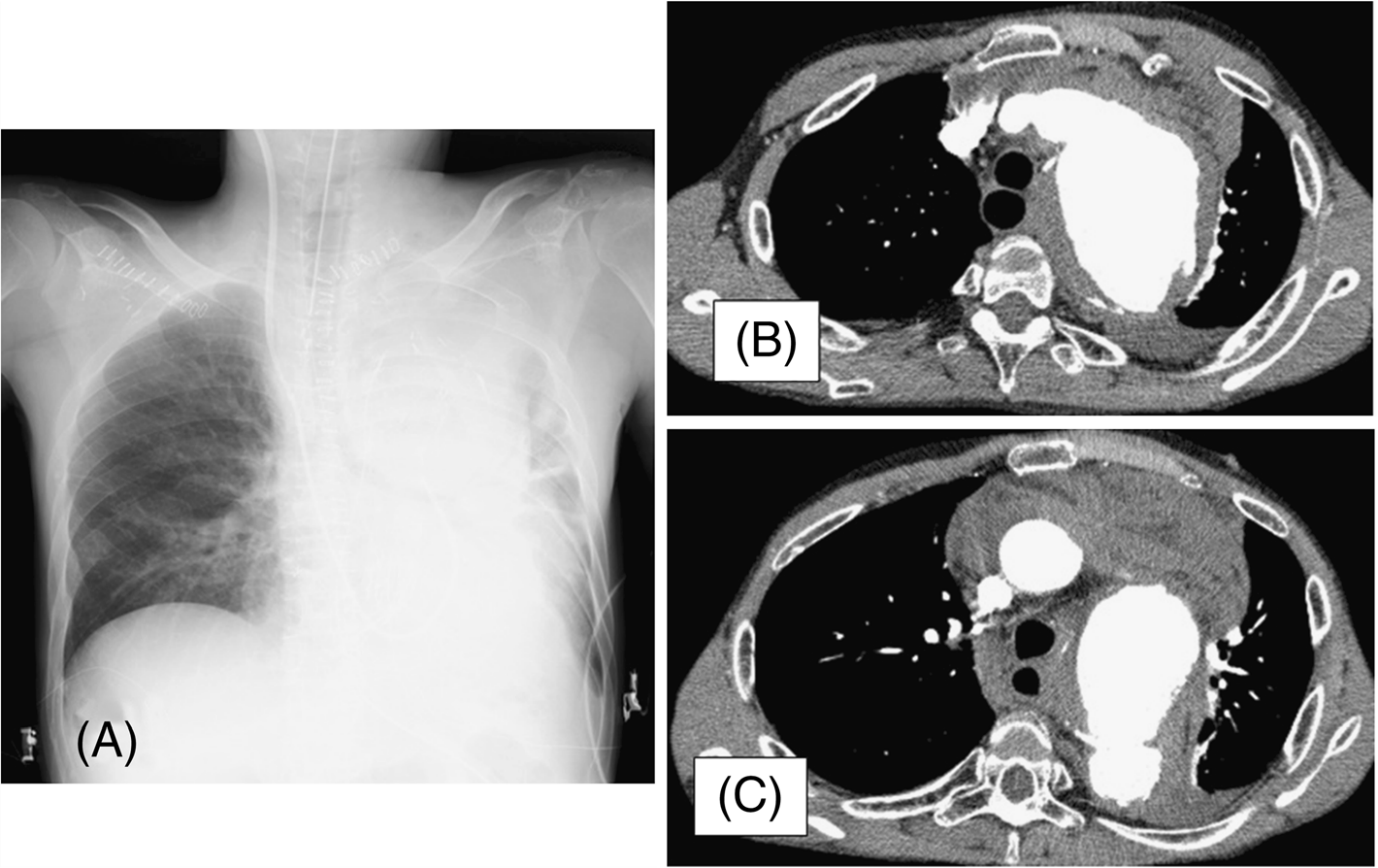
Chest radiograph and computed tomography scan of patient with GPA. (A) Chest radiograph of a patient with a very large aneurysm of the aortic arch. Evident are marked widening of the mediastinum and aortic contour. (B) and (C) Axial contrast-enhanced computed tomography scan of the thorax revealing ruptured aortic aneurysm and collapsed left lung.
